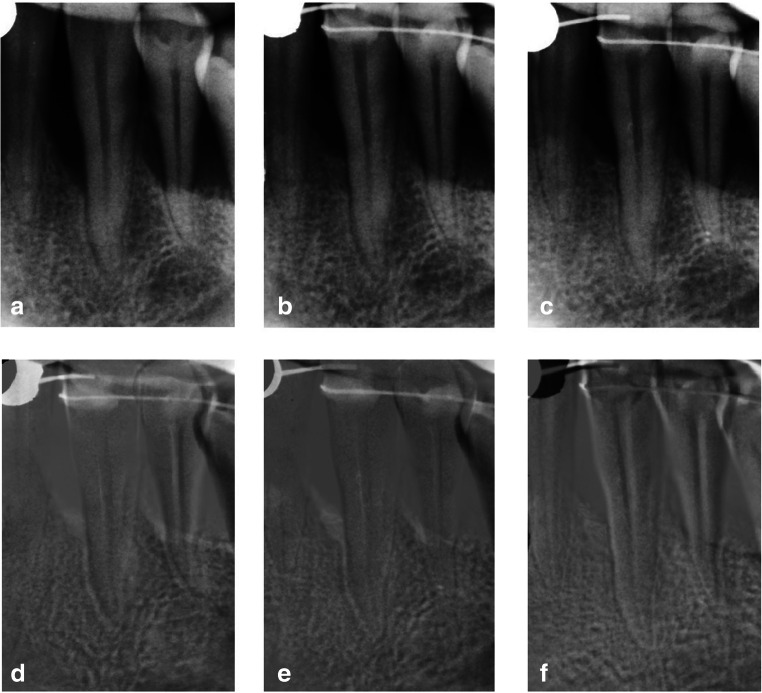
Subtraction radiography of test site (CM-treated). Intrabony defect on mesial surface of tooth 33. a–c Radiographs after normalization. a Baseline radiograph. b Six-month radiograph. c Twelve-month radiograph. d–f Subtraction images. d Subtraction of 6-month radiograph from baseline radiograph. e Subtraction of 12-month radiograph from baseline radiograph. f Subtraction of 12-month radiograph from 6-month radiograph. Minimal density gain as compare between 6 and 12 months post-operatively
